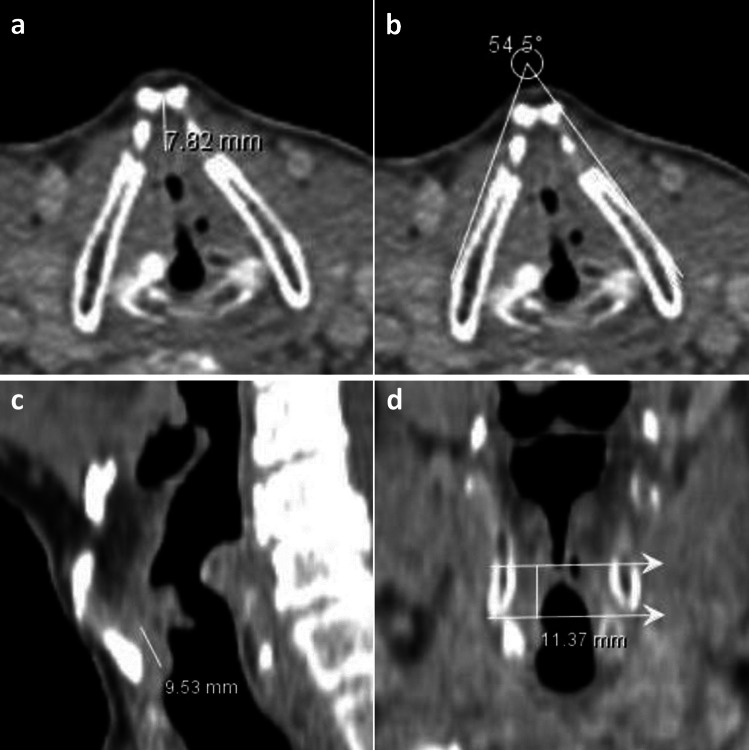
The computed tomography sections supplied depict representative instances of measurements for three parameters associated with the anterior commissure, which are utilized in the radiological assessment of patients involved in the study. These are listed as follows; a Measurement of the thickness of the anterior commissure in the section passing through the anterior commissure in the axial plane, b Measurement of the angle between the two laminae of the thyroid cartilage in the section passing through the anterior commissure in the axial plane, c Measurement of the vertical length of the tumor in the section passing through the anterior commissure in the sagittal plane, d Measurement in the coronal plane of glottic distance (vertical extension ratio was obtained by dividing the measurement in Fig. 1c to the measurement in Fig. 1d)
